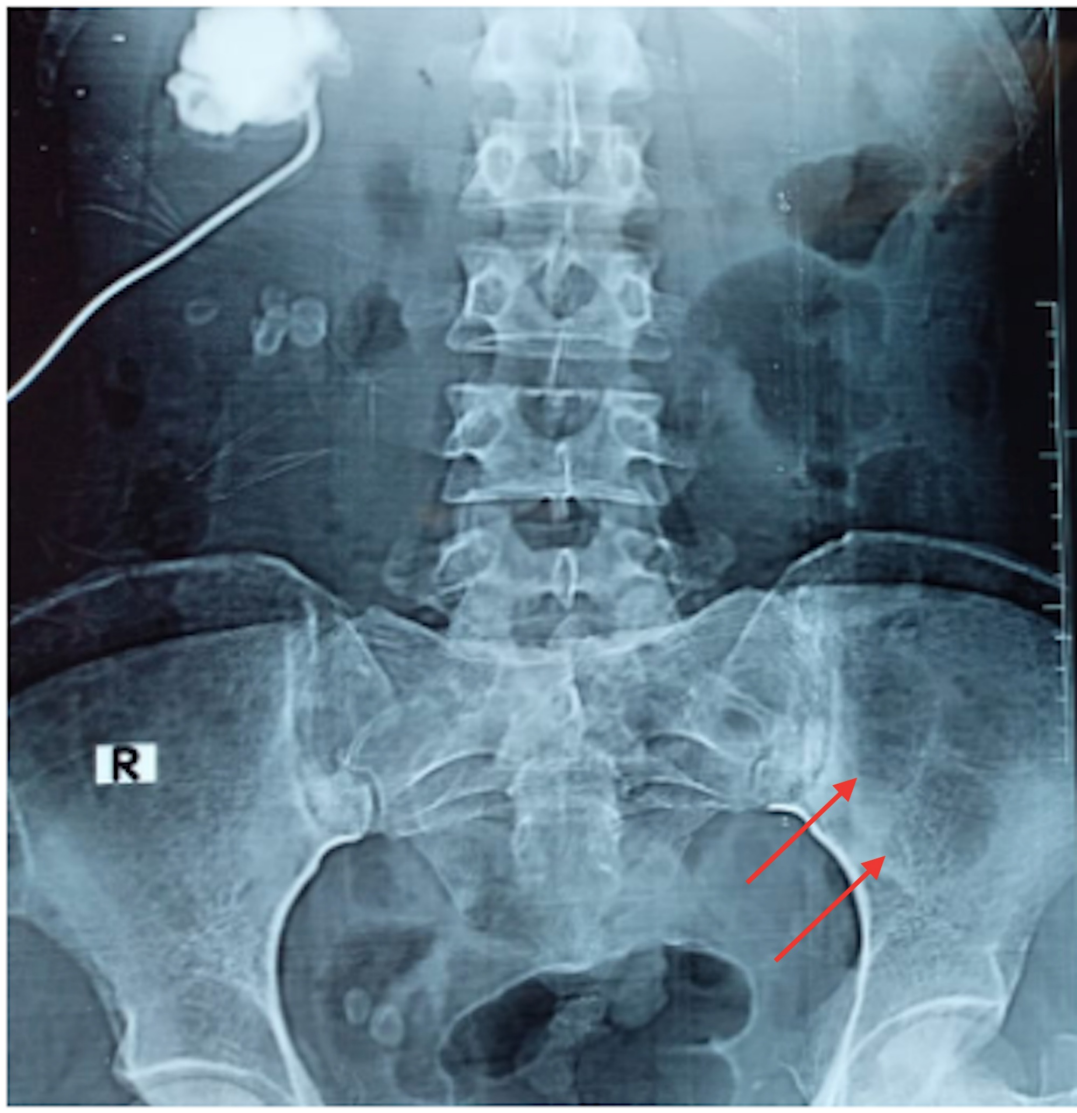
A radiograph of the pelvis showing lytic lesions afflicting the left ileum bone, suggestive of multiple myeloma
